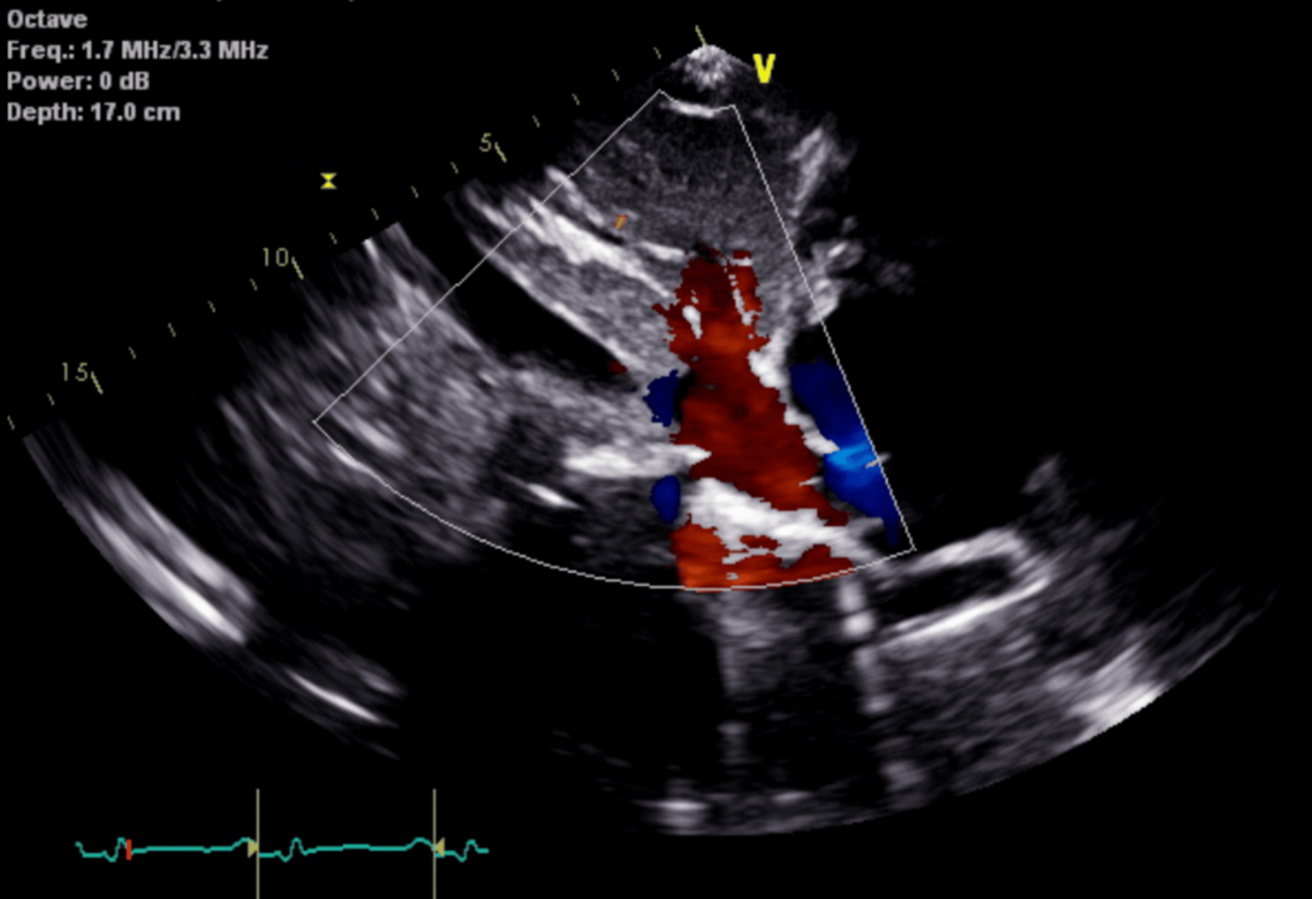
Echocardiogram demonstrates hepatic vein flow reversal, a hallmark of severe tricuspid regurgitation and significant right ventricular overload.
